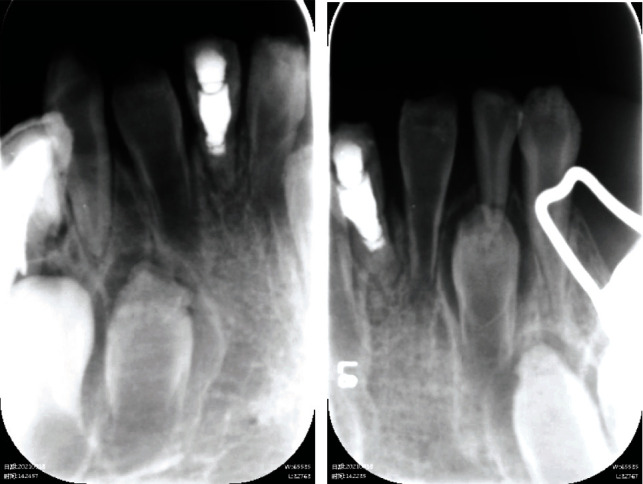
Preoperative radiographs. Short root, thin hard tissue, and enlarged pulp cavity was evident in teeth 31, 41, and 42. Pulp cavity of 41 was filled with radiopaque material. (a) Tooth 42 merely erupted, and periapical radiolucency was noticed. (b) Tooth 31 fully erupted, and periapical radiolucency was seen.
